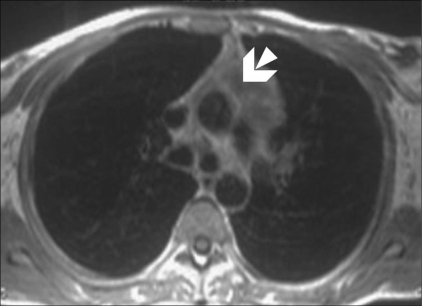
Postcontrast axial T1W MRI image shows peripherally enhancing lymph nodes (arrow) in the prevascular space
